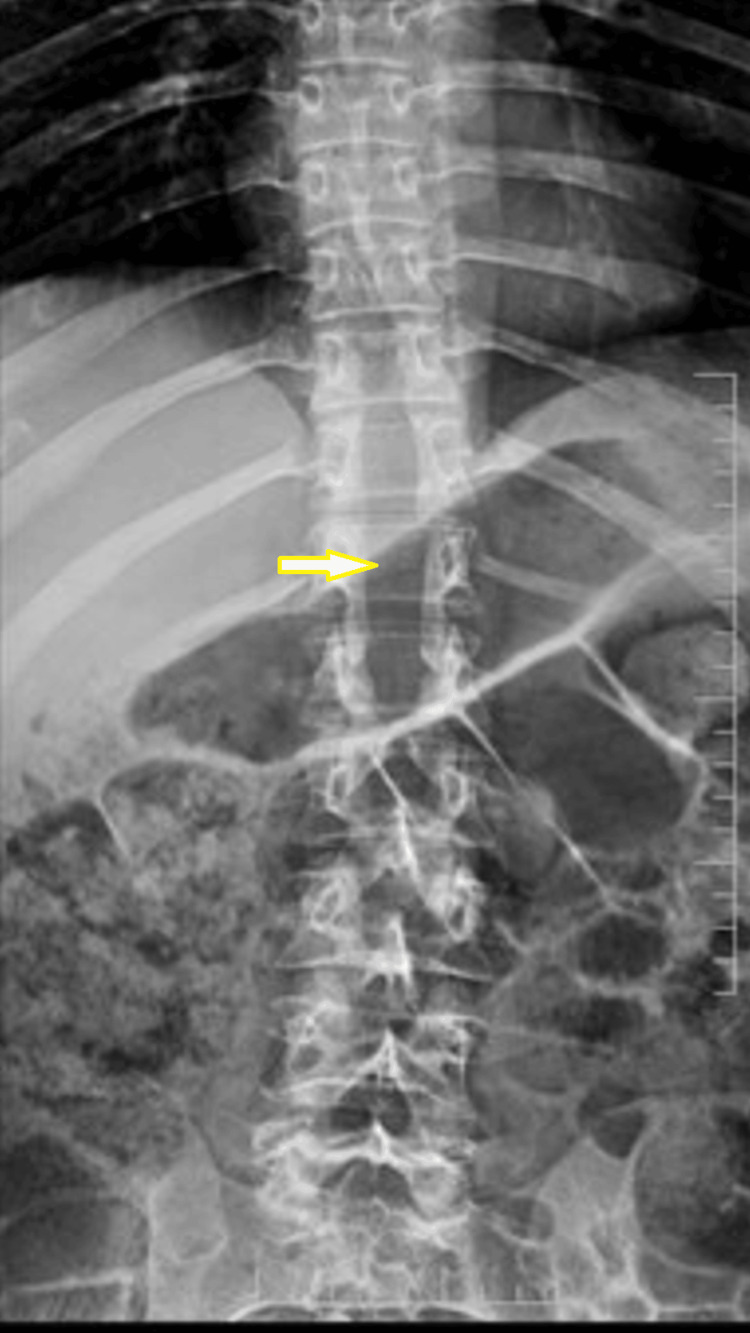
X-ray of the thoracolumbar spine (anteroposterior view) showing the laminectomy window in T9-T12 (yellow arrow) from the previous surgery
